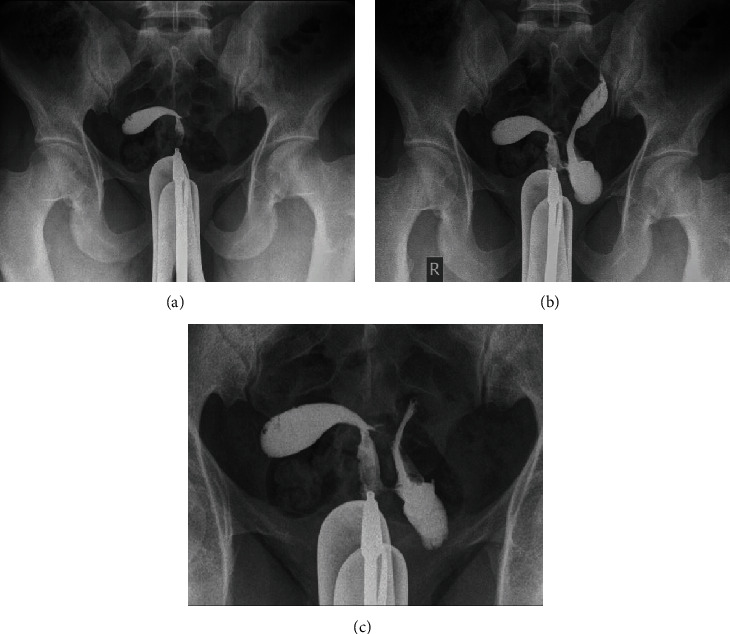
(a) Frontal radiographic just after contrast injection through vaginal cannula: opacification of tubular shape of the endometrial cavity to the right side of the pelvis raising the possibility of unicornuate uterus. (b) Frontal radiographic after few minutes of contrast injection through vaginal cannula: opacification of a tract to towards left side likely at lower cervix or upper vagina and subsequent opacification of second endometrial cavity in the left side. There is also opacification of left hemivagina caudally which is distended due to blind end. (c) Delayed frontal radiograph for evaluation of fallopian tubes. No opacification of fallopian tube is noted in either side.
